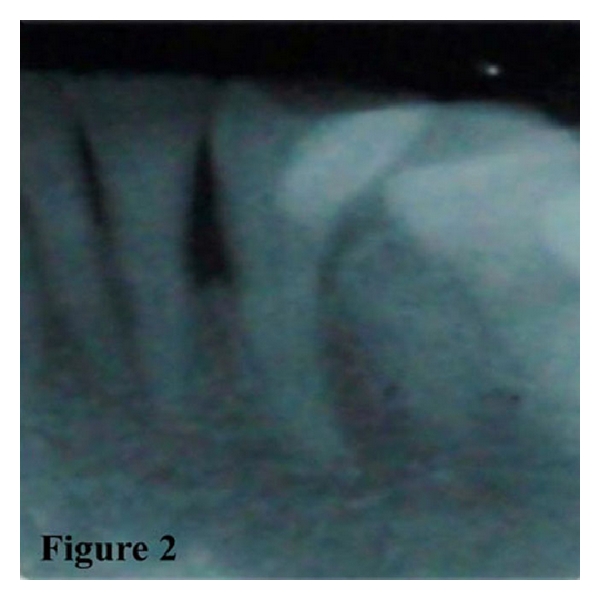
Radiograph showing horizontal bone loss between 32–34 regions.
